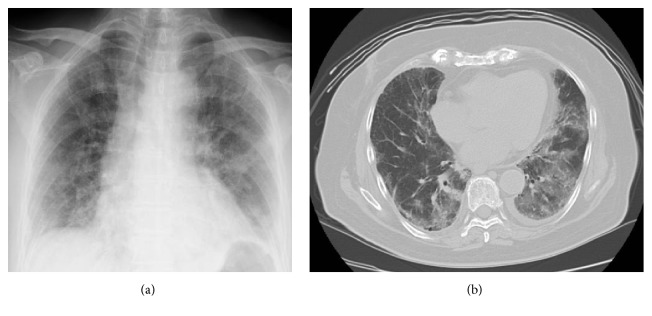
(a) Chest X-ray two months after starting everolimus administration, showing diffuse infiltrative shadows in both lung fields. (b) Chest CT scan two months after starting everolimus administration, showing ground-glass attenuation with diffuse alveolar consolidation in both lung fields.
